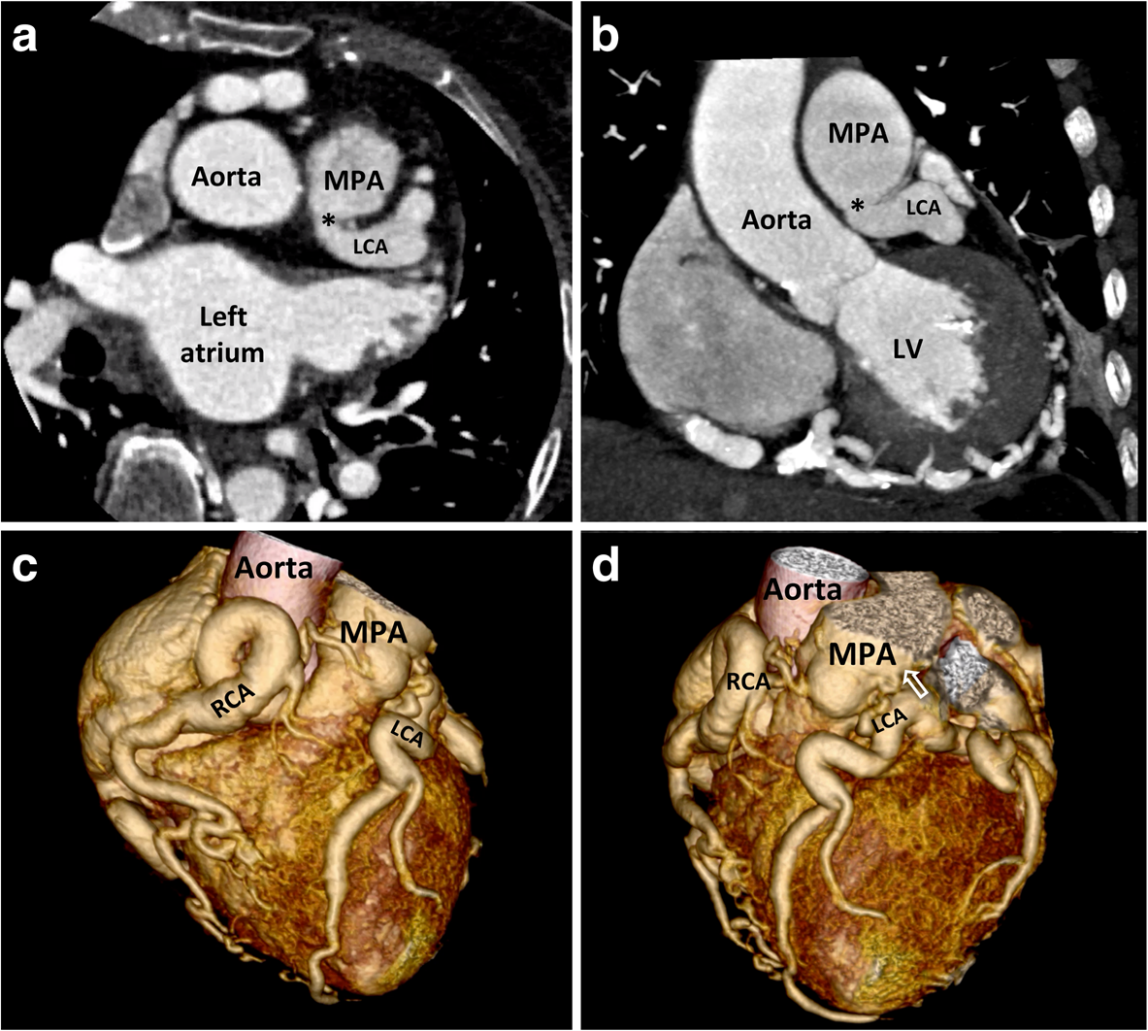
Coronary computed tomography angiography clearly identifies the ALCAPA with a retropulmonary ostium (asterisks, a and b). Volume-rendered image shows the course of the anomalous coronary arteries along the epicardial surface and where the LCA connects to the MPA (c, open arrow, d). Abbreviation as Figs. 1 and 2
